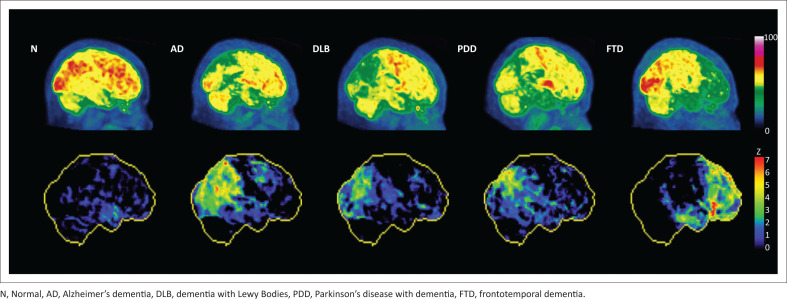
Fluorine-18 FDG PET scans in dementia. Maximum intensity projections of the PET scan (in the right lateral position) are shown on the top row (individually scaled for comparability). Corresponding statistical images (reductions compared to a normal database) are shown in the bottom row.
